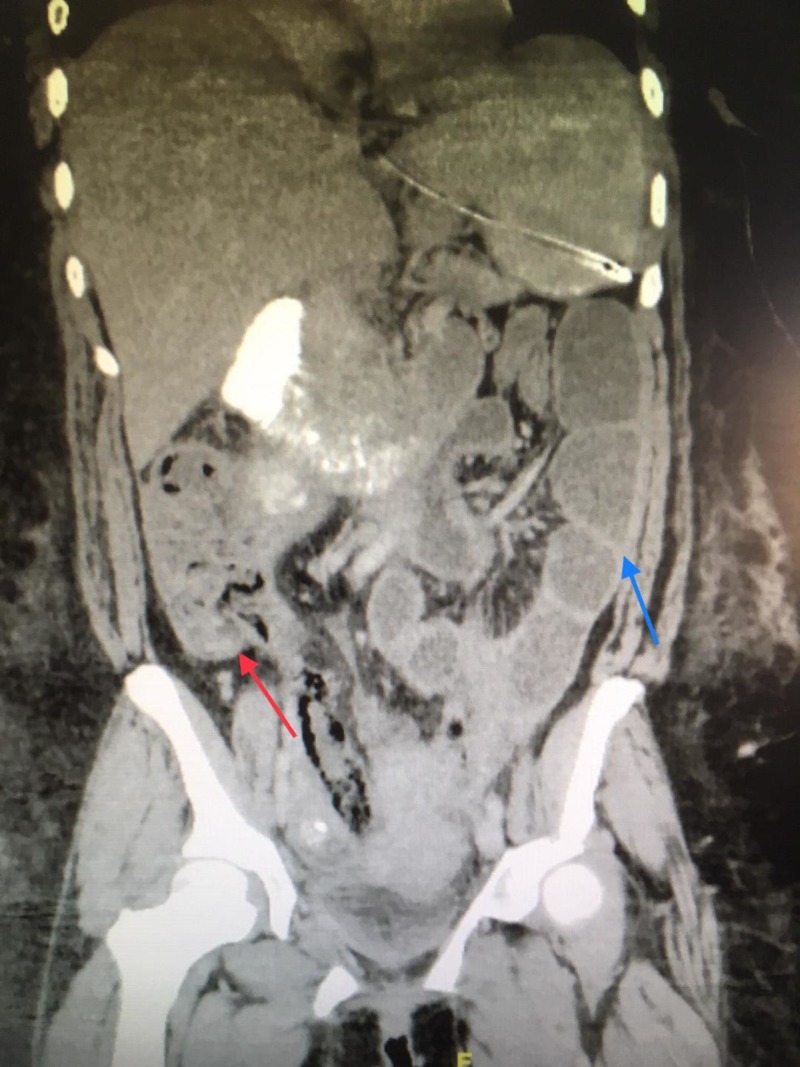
Computed tomography of the abdomen and pelvis Raising more concerns for ischemic bowel. Red arrow: thickened colon wall; blue arrow: thickened small bowel wall
